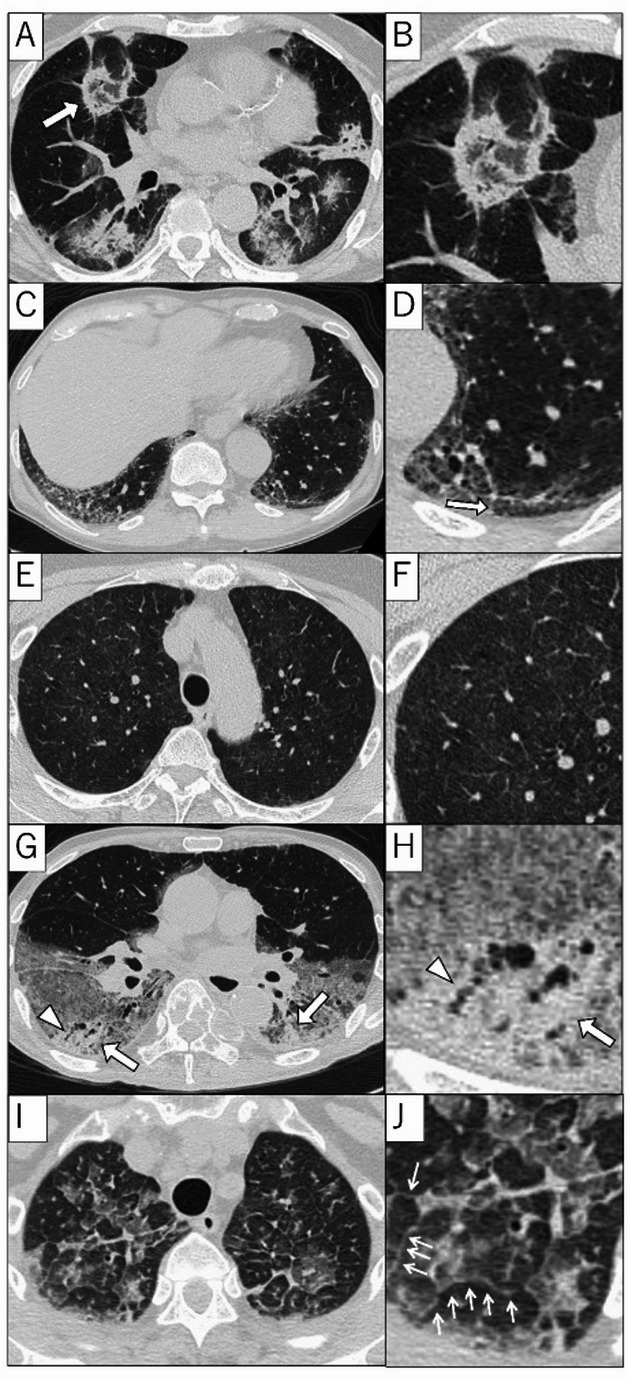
Representative high-resolution CT (HRCT) patterns of Drug-induced lung injury. A, B Organizing Pneumonia (OP) pattern. Multiple ground-glass opacities (GGOs) and consolidation are seen. Areas with GGO surrounded by a rim-like consolidation (reversed-halo sign, arrow, A) are also seen. C Nonspecific Interstitial Pneumonia (NSIP) Pattern. There are GGOs and mild retraction with peripheral predominance on the HRCT. The subpleural regions are relatively spared (subpleural sparing). D Enlarged image of subpleural sparing (arrow). E Hypersensitivity Pneumonitis (HP) Pattern. Bilateral diffuse, ill-defined centrilobular nodules/ GGO are seen on HRCT. F Enlarged image of ill-defined nodules/ GGO. G Diffuse Alveolar Damage (DAD) Pattern. HRCT image shows extensive GGOs and consolidations (arrows) with dependent zone predominance. Traction bronchiectasis (arrowhead) is also seen. H Enlarged image of consolidation (arrow) and traction bronchiectasis (arrowhead). I Acute Eosinophilic Pneumonia (AEP) pattern. Bilateral diffuse GGO and interlobular septal thickenings are seen on HECT. J Enlarged image of GGO and interlobular septal thickenings (arrow)
